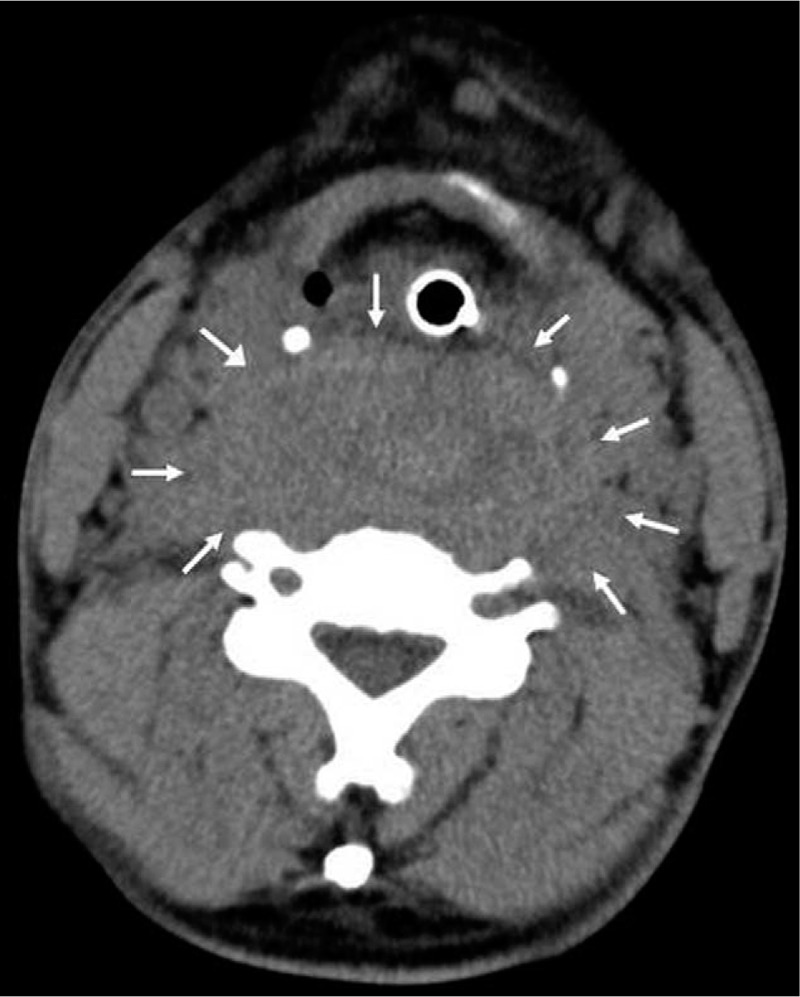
Post-intubation computed tomographic image of the neck. Axial view shows a retropharyngeal hematoma occupying the whole retropharyngeal space (arrows) and the endotracheal tube maintaining airway patency.
